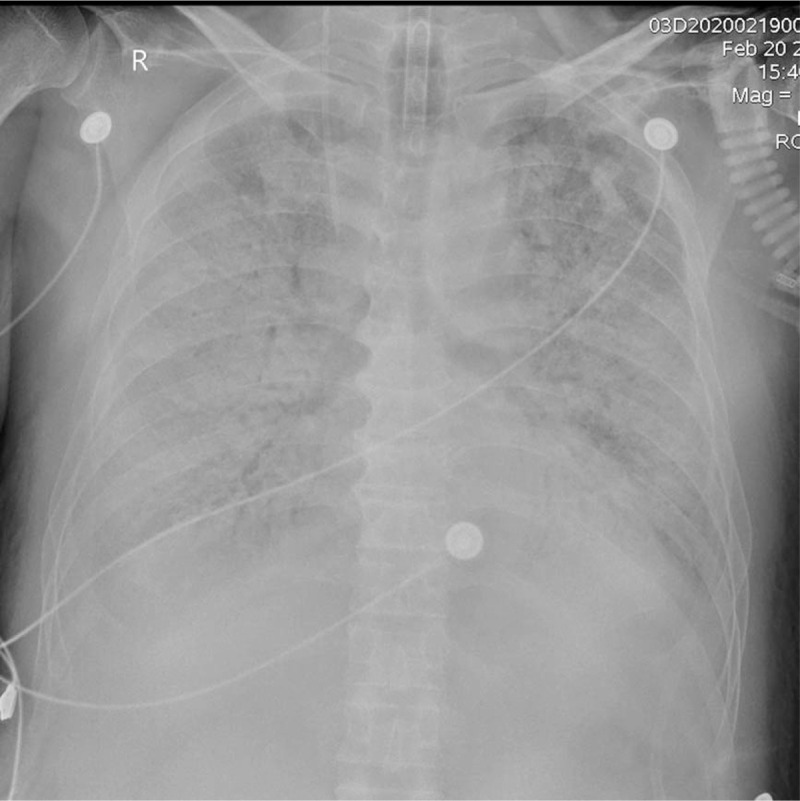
Male, 69 years old. There were multiple areas of opacification in both lungs, showing diffuse high-density patchy opacity with uneven density, presenting “white lung.” The patient died 4 hours later after the X-ray.
